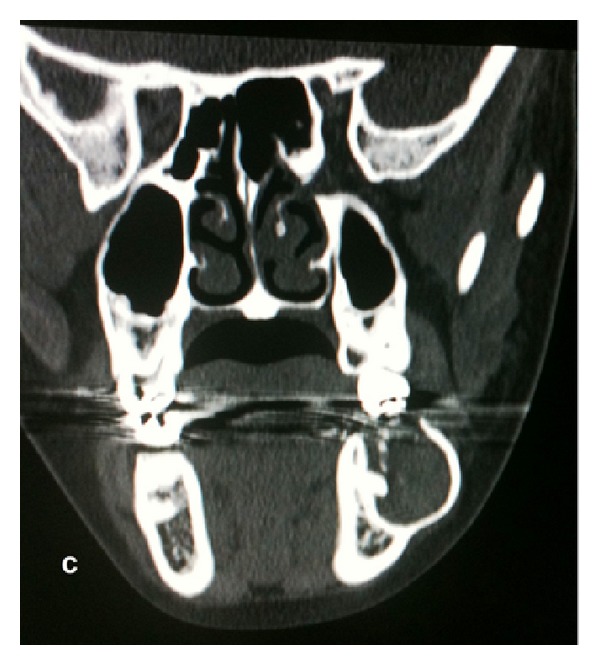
Coronal CT showing a hypodense lesion, containing irregular hyperdense areas in its inside, associated with the expansion of the vestibular cortical bone and the resorption of the cortical bone of the alveolar process.
